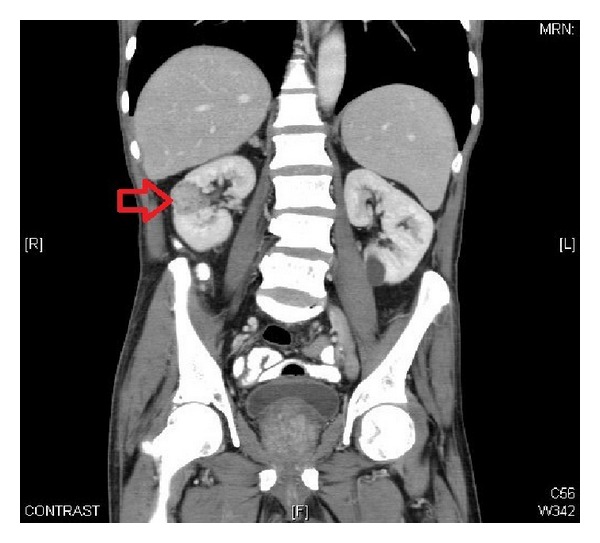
Computed tomography of abdomen (coronal view) showing right kidney mid pole mass turned out as renal cell carcinoma upon radical nephrectomy.
